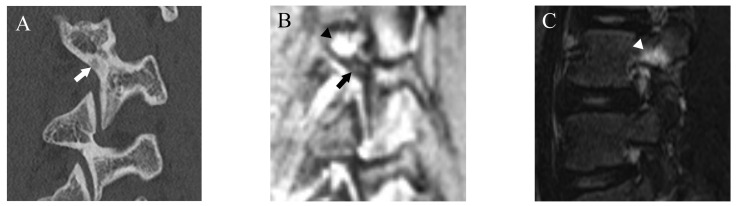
Representative images of grade 1 fracture. The right pars interarticularis of L3 from a 14-year-old male. (A) Oblique-sagittal CT image showing faint attenuation in the cortex (arrow). (B) The faint attenuation corresponds to slight hyperintensity in the cortex on an oblique-sagittal DESS image (arrow). The window of the image was adjusted to enhance the faint finding. Note the BME in the pedicle (arrowhead). (C) Sagittal STIR image shows BME in the right pedicle of L3 (arrowhead).
